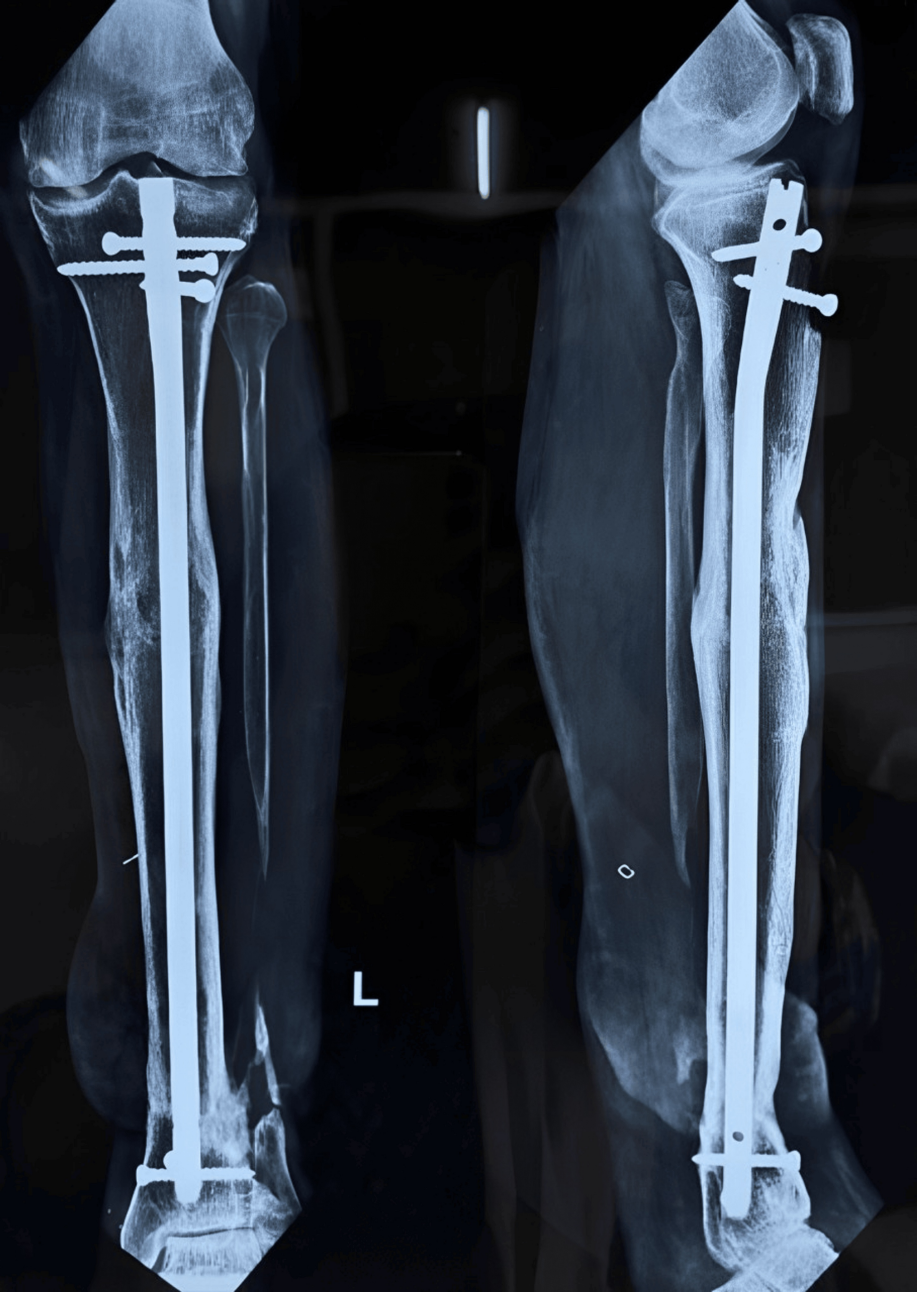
X-ray done at 12 months showing the complete union at the fracture site
